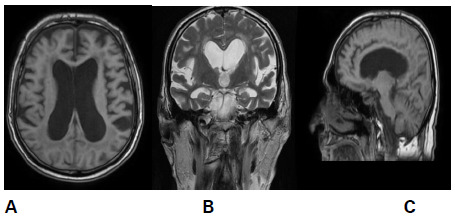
A) MRI: T1 weighted image shows dilated lateral ventricles, B) Coronal T2 weighted imaging shows acute callosal angle, C) Sagittal T1 weighted image shows upward bowing of corpus callosum.
